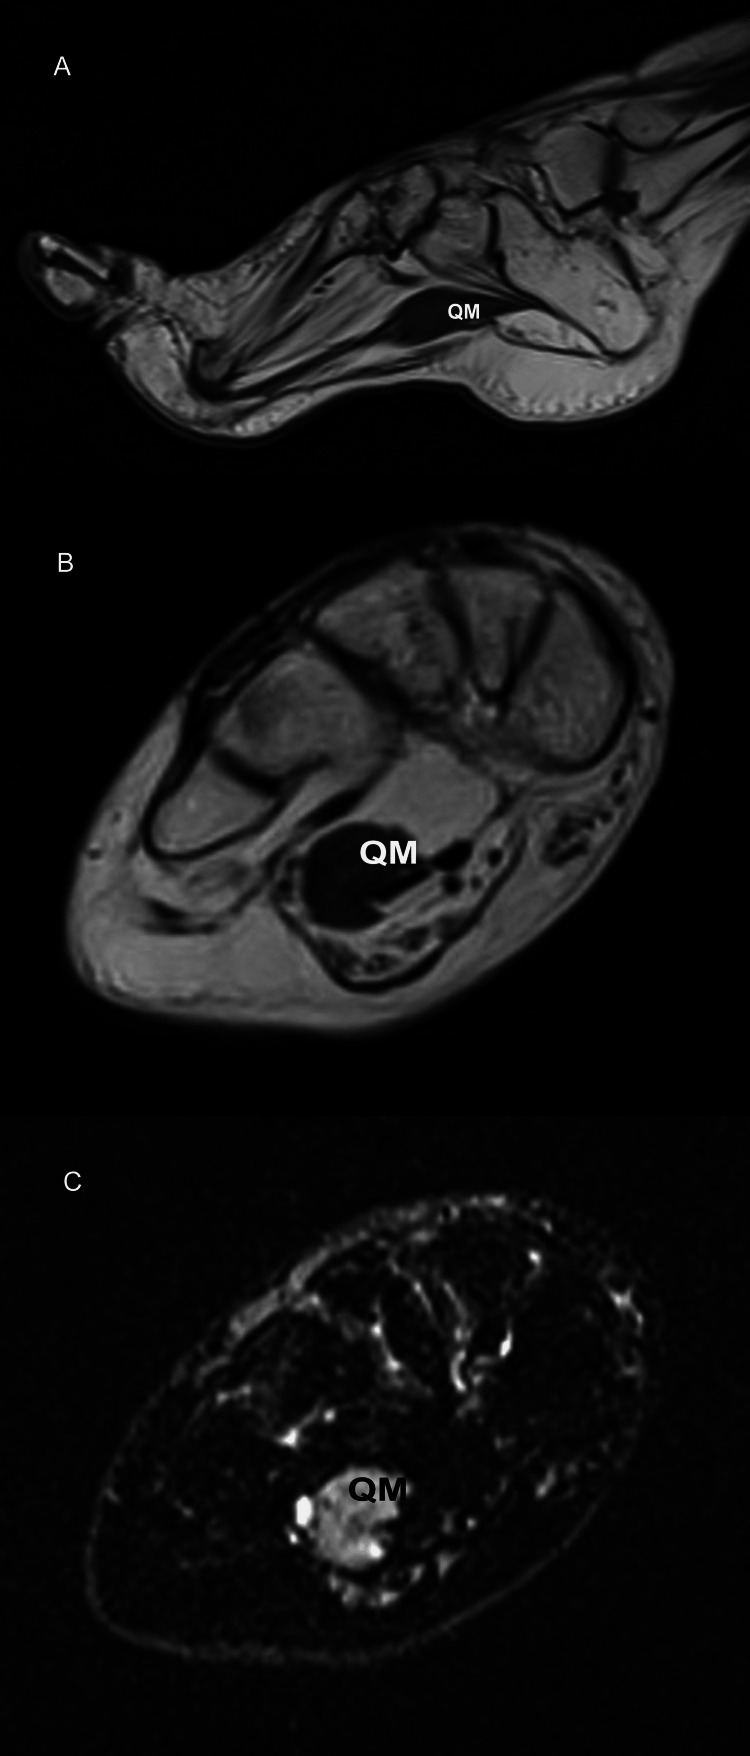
A 46-year-old female refugee with foot drop related to skeletal muscle channelopathy (sodium channel myotonia). (A) Sagittal T1-weighted MR image shows pes cavus deformity with severe, gross fatty atrophy of intrinsic foot muscles. The quadratus plantae muscle (QM) is spared. Axial T2-weighted (B) and short-tau inversion recovery (C) MR images show QM of intermediate T2-weighted and predominant high signal intensity on short-tau inversion recovery images. Abnormal increased inward flux of sodium in muscle cells may account for edema-like changes in the quadratus plantae muscle.
